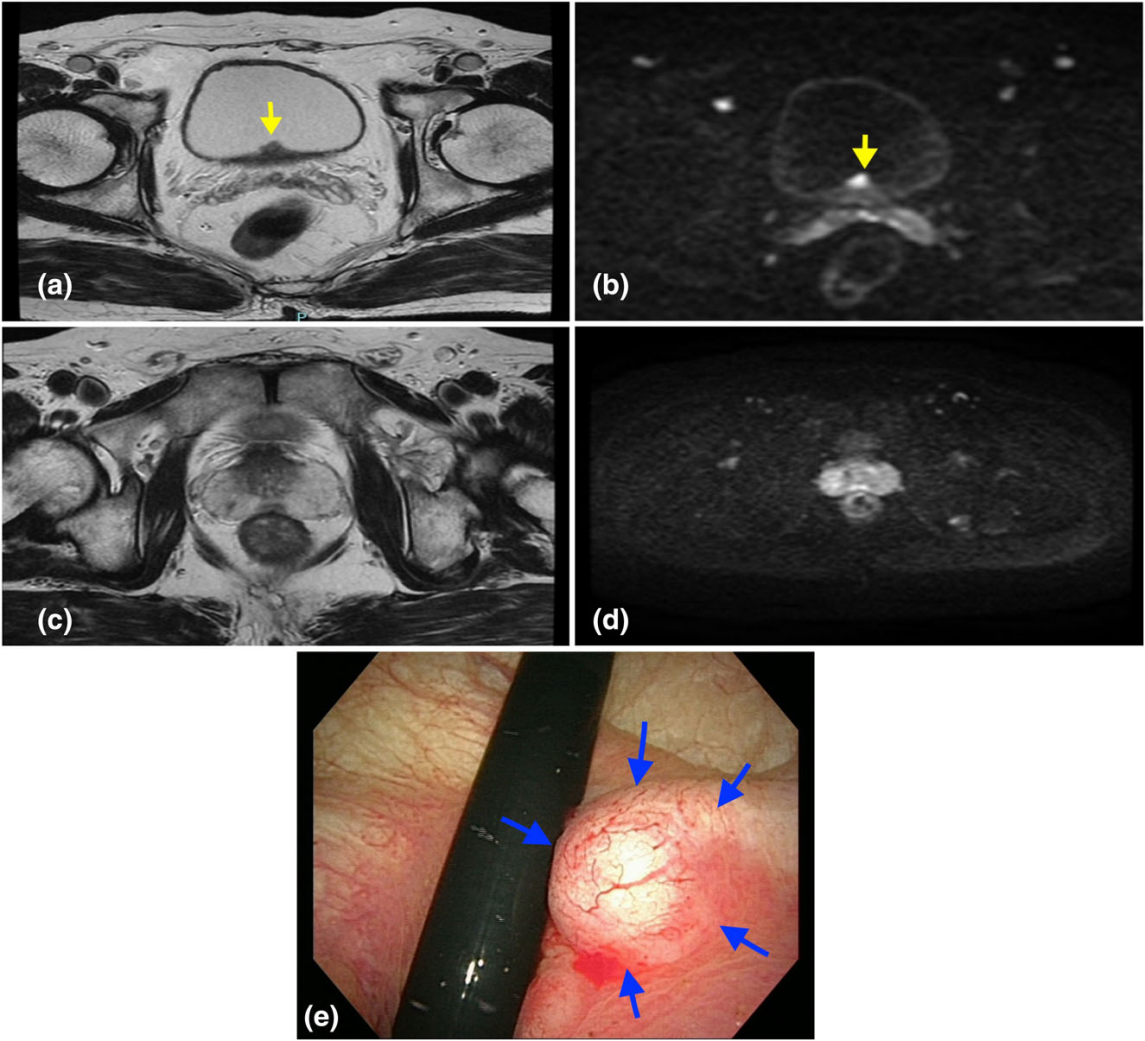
(a–e) Abdominal MRI and Cystoscopy image. (a) T2‐weighted imaging and (b) diffusion‐weighted imaging of the bladder. The yellow arrow indicates a nodular‐type tumor in the bladder trigone. (c) T2‐weighted imaging and (d) diffusion‐weighted imaging of the prostate, which show an area suspected to be PCa in the peripheral zone of right lobe of prostate. (e) Cystoscopy image showing a submucosal tumor in the bladder trigone (blue arrows).
